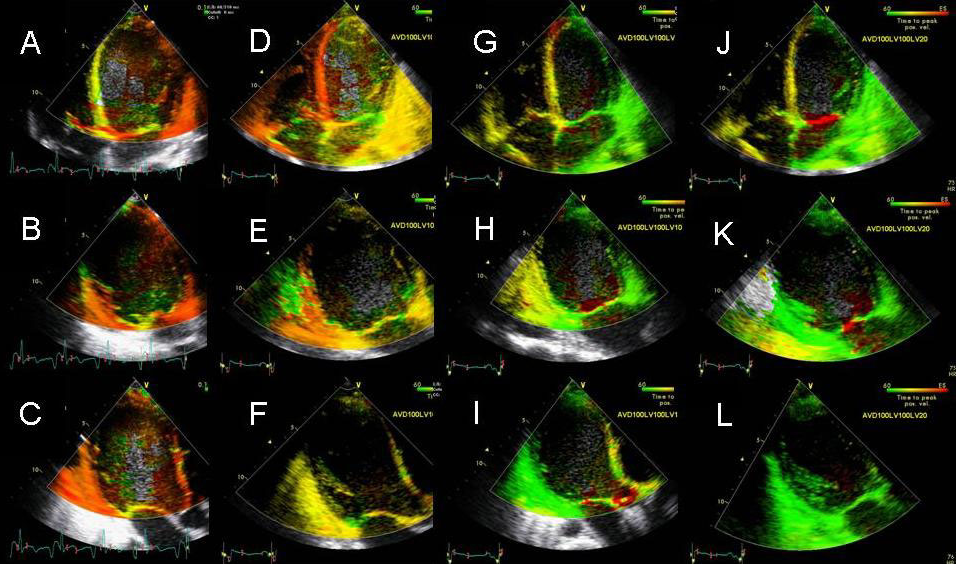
TSI in an 18 year old male with Coxsakie B virus cardiomyopathy and narrow QRS. Significant mechanical dyssynchrony was identified on TSI in the apical 4 (A), 2 (B) and 3 chamber (C) views (red color in the myocardium). Panels D-F are same views post CRT at AVD of 100 and VV delay of 0 ms. LV pre-excitation by 10 ms is shown in panels G-I and by 20 ms in panels J, K and L respectively. Note persistence of mechanical dyssynchrony post CRT (D-F) with worsening of right ventricular delay and mild improved in LV delay. Progressively decreased intra as well as interventricular dyssynchrony occurred with increasing LV pre-excitation (G-L).
